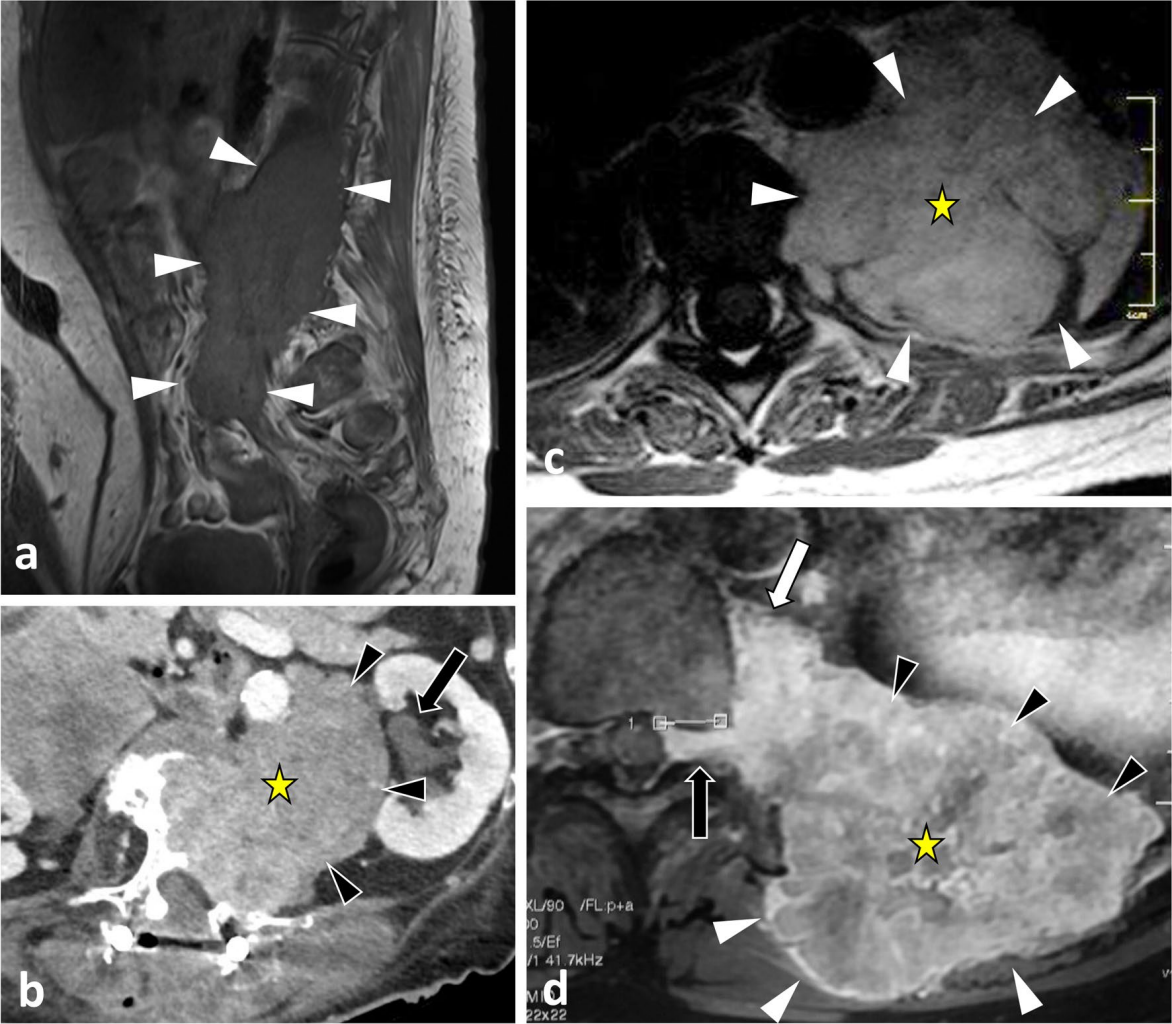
Longitudinal and transversal extension of three distinct paraspinal tumours. Spinal and paraspinal metastasis* of urothelial carcinoma of the left upper tract in an 83-year-old female (a, b). Sagittal T1WI a shows the longitudinal extension of the tumour within the left psoas muscle from L2 to S1 level. Contrast-enhanced axial CT scan reconstruction (b) demonstrates the transversal extension of the mass within the retroperitoneum (arrowheads), causing ureteral stenosis (black arrow). *Undifferentiated sarcoma* of soft tissue in a 37-year-old female (c). Contrast-enhanced axial T1WI (c) shows pleural extension (arrowhead). *Costal Ewing’s sarcoma* in a 23-year-old male (d). Contrast-enhanced axial T1WI (d) shows an extension in epaxial muscles (white arrowheads), a pleural and intercostal extension (black arrowheads), a posterior mediastinum extension (white arrow) and an epidural extension via the intervertebral foramen (black arrow). Yellow stars show the epicentre of masses
